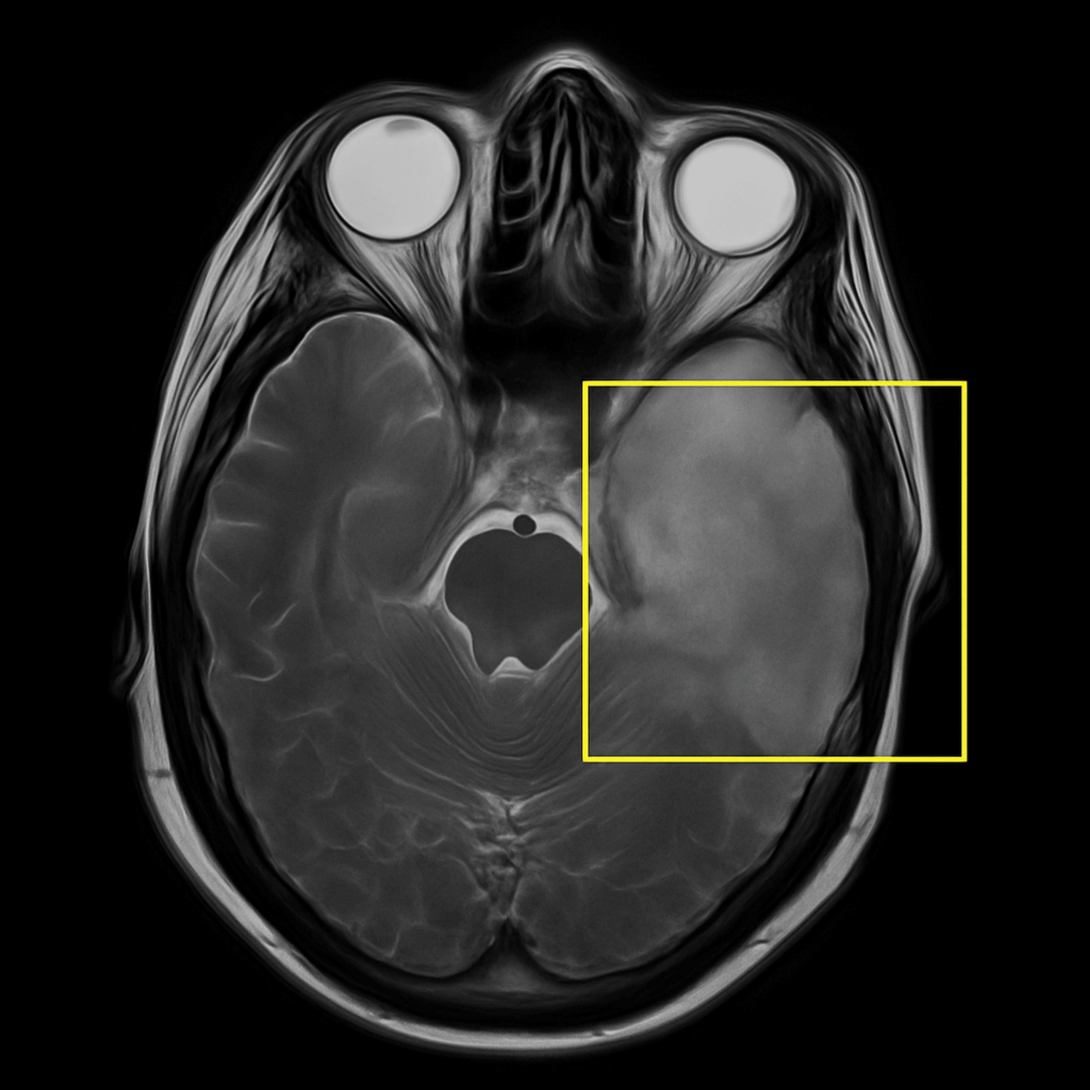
Axial T2-weighted MRI showing hyperintense signal in the left temporal lobe with loss of gray–white differentiation, consistent with herpes simplex encephalitis
